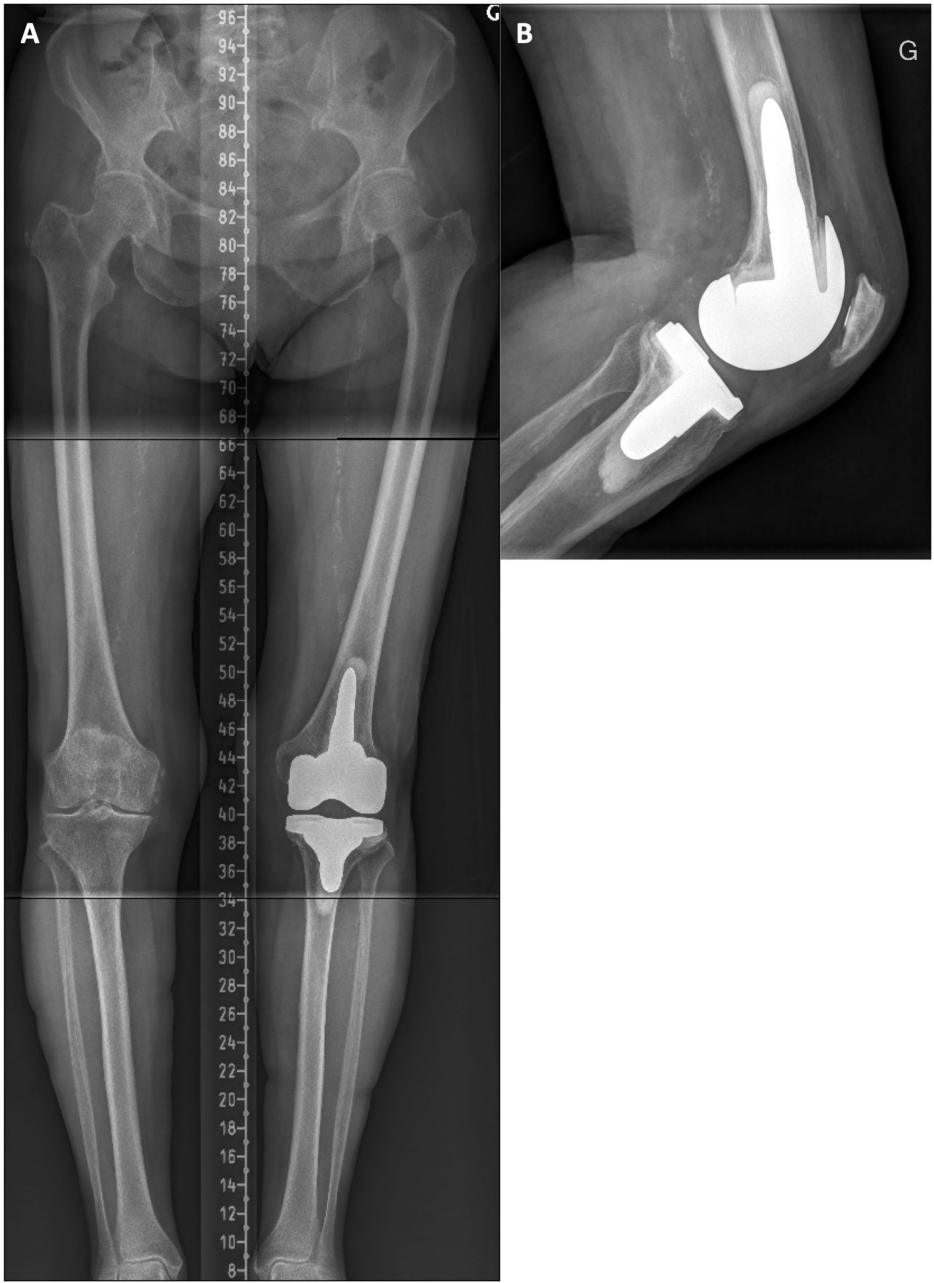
(A) Post-revision standing long-leg X-ray of the left lower limb where the implant mDFA has been modified to 2.0° valgus and the mPTA at 3.0° varus (1.0° varus aHKA). As expected, the femoral cemented stems is not aligned with the femoral bone diaphysis. On the tibial side, to restore patient's alignment, only a stubby stem could be used and comes in contact with the lateral cortex. (B) Left knee lateral view where the implant posterior tibial slope has been reduced to 3.0°. Because manufacturer recommendations for this implant is a neutral slope, we did not aim at patient's contra-lateral side value of 6°.
